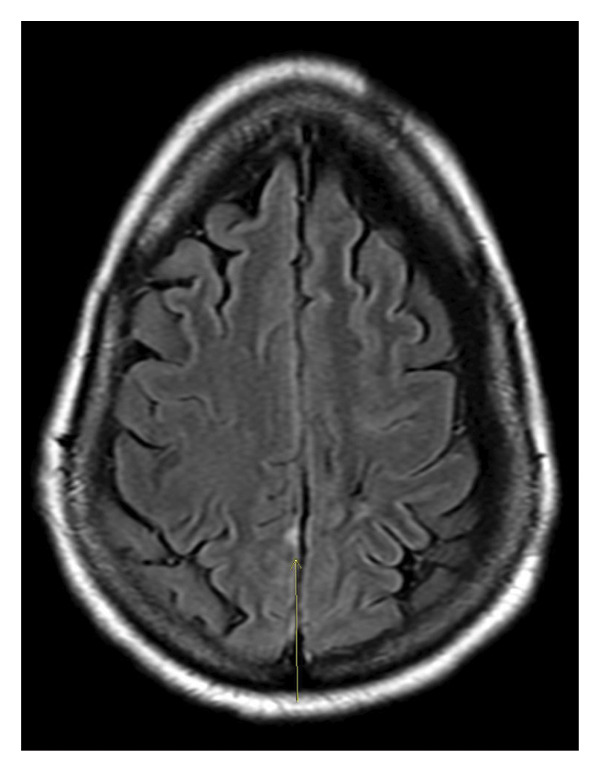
MRI brain, axial T2 FLAIR sequence (image brightness adjusted to optimize visualization).
